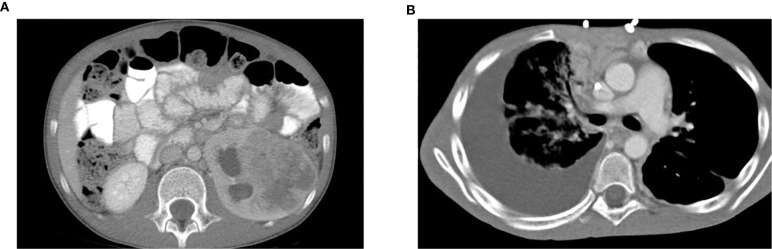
A patient presents with a large left renal mass and a subsequent large right pleural effusion. (A) CT scan of the abdomen on initial presentation showed a left renal mass measuring 6.8 x 5.1 x 7.3 cm with diffuse necrotic change. No involvement of the right kidney was identified. (B) CT scan of the lung six months later showed a rapidly developed large right PE. Worsening of pulmonary metastases with development of lymphangitic carcinomatosis was also noted.
